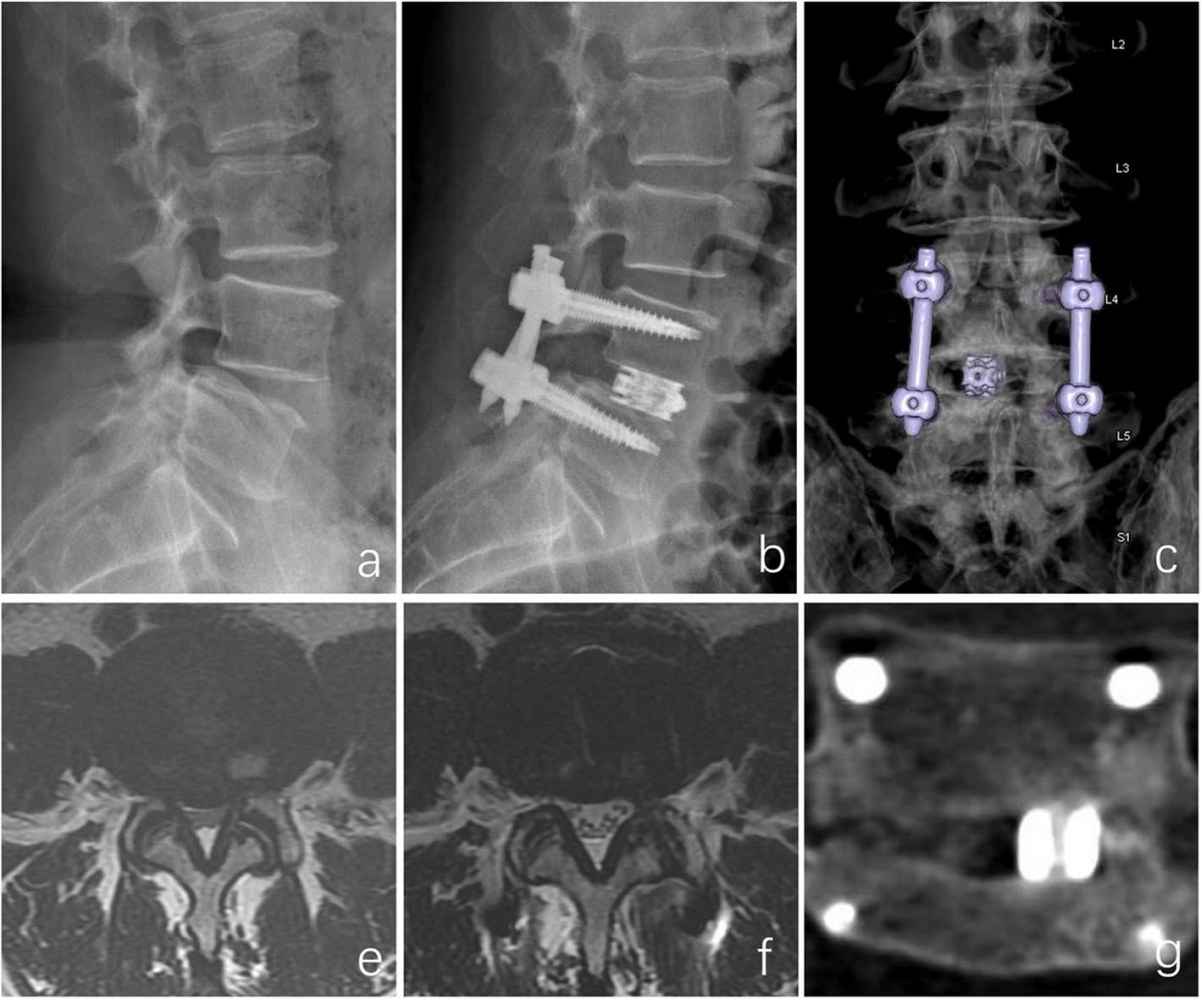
A 57-year-old woman complained of low-back pain and left lower limb pain and numbness. Preoperative lateral X-ray (a) shows spondylolisthesis (I°). Postoperative lateral X-ray (b) shows complete resolution of the spondylolisthesis and normal disc height. Reconstructed CT image (c) shows the cage position and the range of articular process molding. Axial MRI (e, f) shows significant improvement in the spinal canal and complete nerve decompression. Coronal CT (g) at the last follow-up shows definite interbody fusion
